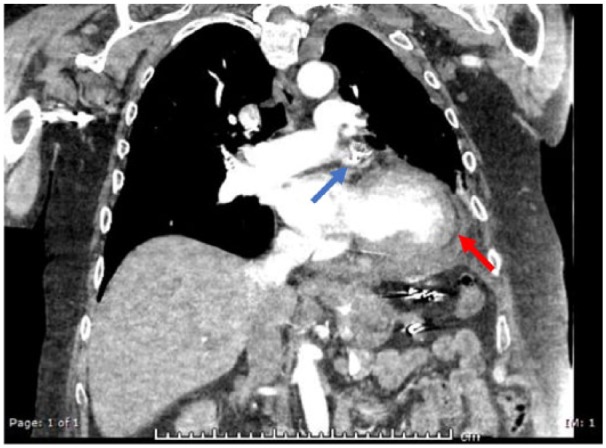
Computed tomography chest 1-month postprocedure showing adequate device positioning (blue arrow) with a small residual pericardial effusion (red arrow).
